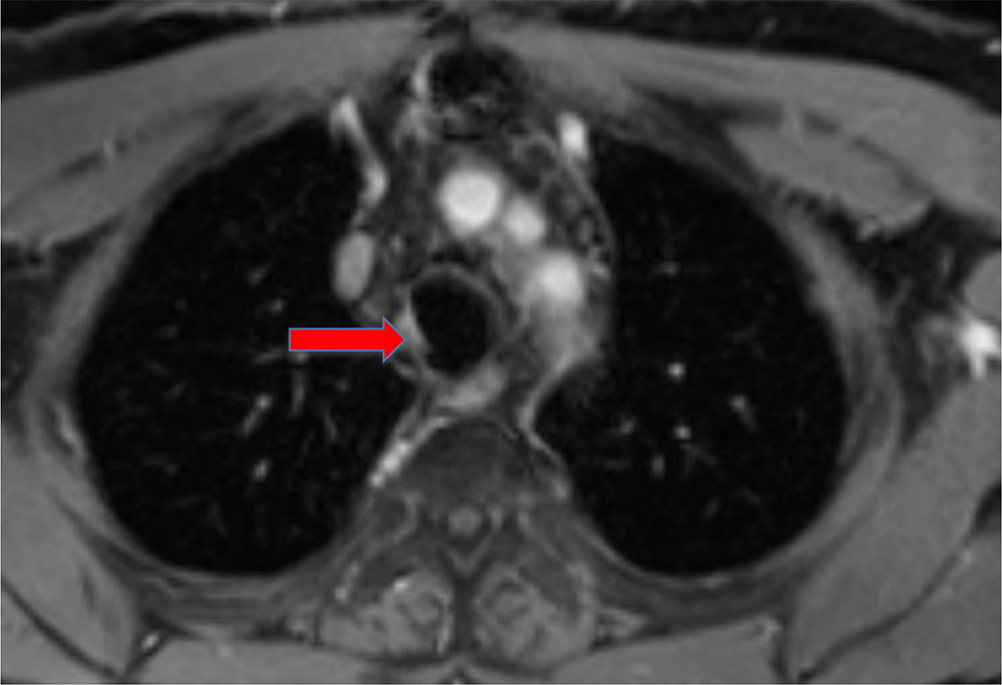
Contrast-enhanced MRI of the mediastinum: axial slice. Block arrow: further reduction in tumour size, 3 years post-treatment (November 2014).
